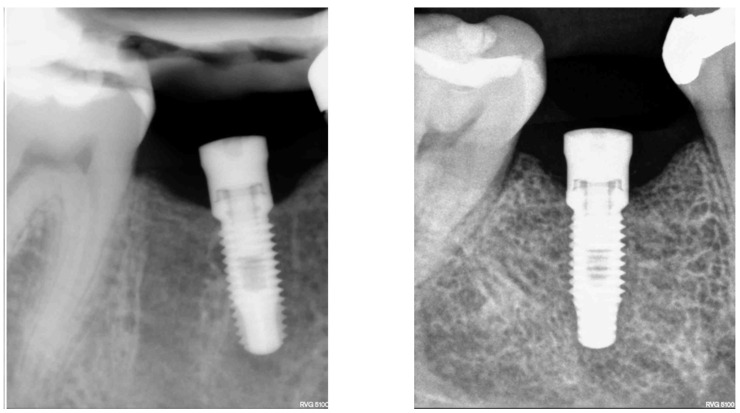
Radiographic aspect showing absence of peri-implant bone loss.
